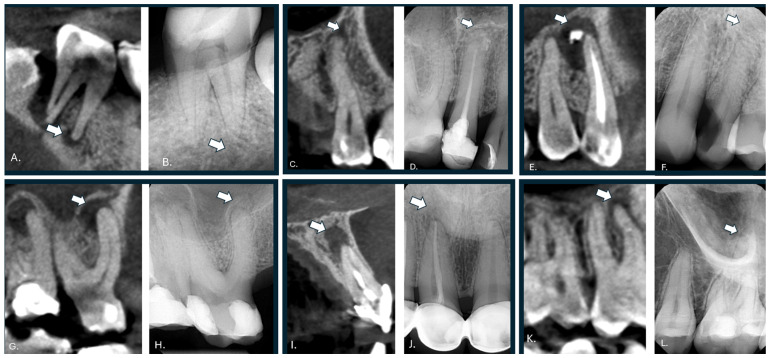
This figure presents tooth CT images (A,C,E,G,I,K) and RVG (B,D,F,H,J,L) in pairs, where arrows indicate lesions for which visualization was uncertain for medical professionals but evident on CT images.
